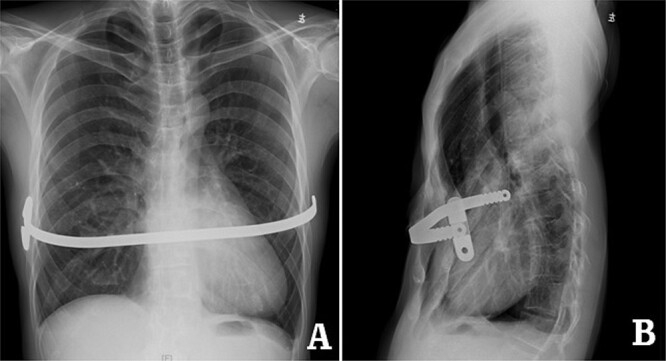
X-ray examination before operation. (A) Posteroanterior radiograph; and (B) lateral radiograph.
